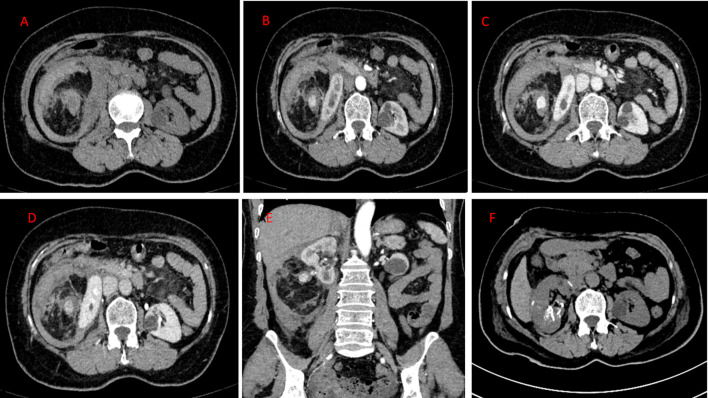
Preoperative and postoperative CT images of the lower abdomen + pelvis with contrast enhancement in patients with renal angiomyolipoma. (A) Preoperative non-contrast CT image showing a renal space-occupying lesion; (B) Preoperative arterial phase CT image showing uneven enhancement in the lesion area; (C) Preoperative venous phase CT image showing changes in the enhancement degree of the lesion over time; (D) Preoperative excretory phase CT image displaying the anatomical relationship between the lesion and the renal pelvis and calyces; (E) Preoperative coronal reconstruction CT image during the arterial phase, clearly showing the spatial location and extent of the lesion; (F) Postoperative follow-up CT image showing good structural recovery of the affected kidney with no significant hematoma or residual lesions.
